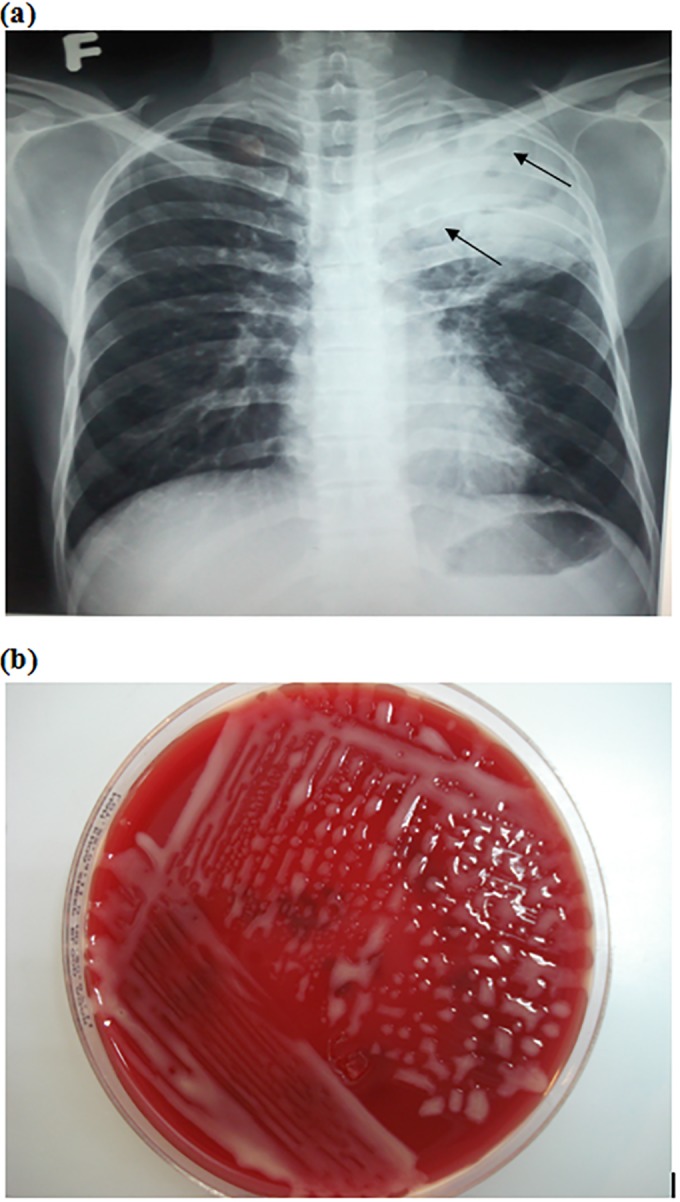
(a) Chest X-ray of patient 1 with arrows indicating several cavitary lesions within the left upper lobe consolidation; (b) salmon-pink colonies after 7-day incubation of the pleural fluid, plated on Colombia blood agar.
